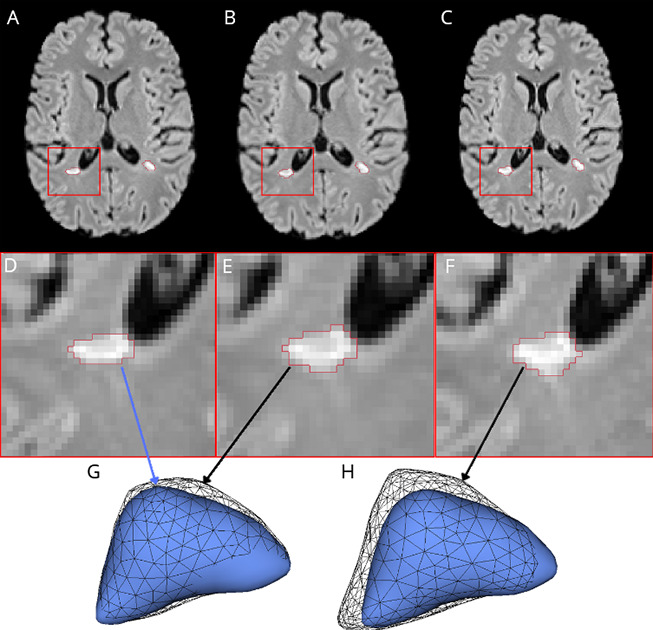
MRI-defined slowly expanding lesions (SELs) based on segmentation analysis. FLAIR MRI axial slice and T2 lesions contoured in red at (A) baseline, (B) 12-month follow-up, and (C) 24-month follow-up, all rigidly registered to an undisplayed reference scan. A region of interest corresponding to the red box, with an MS lesion at its center at (D) baseline, (E) 12-month follow-up, and (F) 24-month follow-up. A 3D representation of the evolution of the MS of the region of interest, with the blue surface being the lesion at baseline (volume 211 mm^3^) and the black wire frame being the lesion at (G) 12-month follow-up (volume 254 mm^3^) and (H) 24-month follow-up (volume 290 mm^3^).
